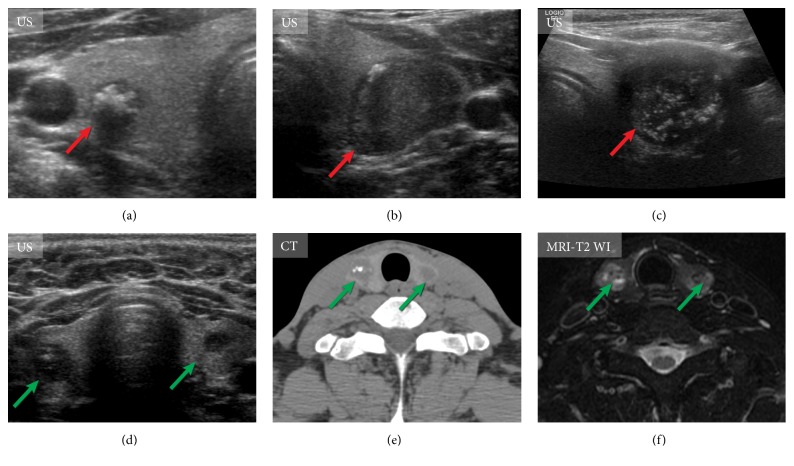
Imaging of the primary tumor in medullary thyroid carcinoma. (a)-(c) Ultrasound (US) features of primary medullary thyroid carcinoma of different patients (red arrows). US of the thyroid gland shows markedly hypoechoic nodules with marked calcifications. (d)-(f) US of the neck, computed tomography, and magnetic resonance tomography (T2 weighted images of the neck) of the same patient with bilateral medullary thyroid carcinomas (green arrows).
